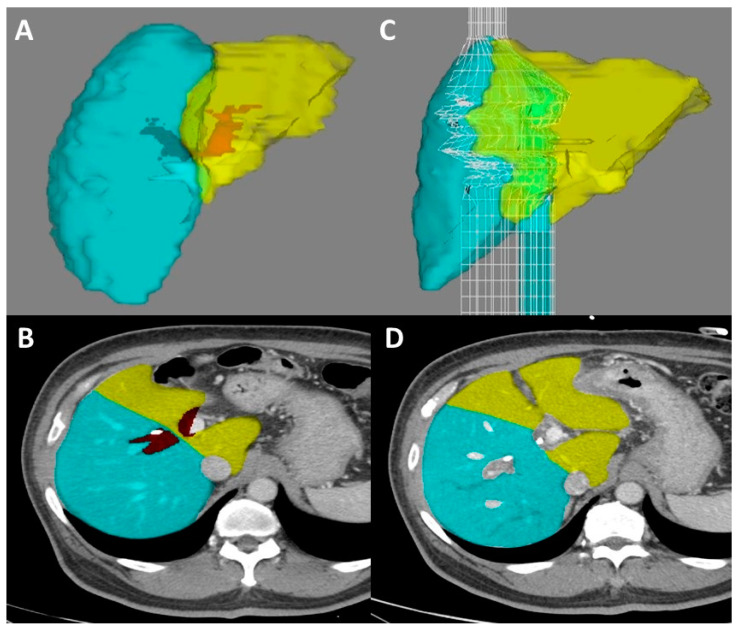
Preoperative and postoperative volumetric analysis with interval portal vein embolization. Computed tomography-based volumetric analysis (TeraRecon Inc., Durham, NC, USA) with perihilar cholangiocarcinoma. Prior to portal vein embolization (panels (A,B)), functional liver remnant after planned right hepatectomy was calculated to be 31.9% (yellow). Patient underwent right portal vein embolization without embolization of Segment IV. After three weeks, repeat volumetry was performed (panels (C,D)). The newly calculated FLR (yellow) was 45.9%, yielding a KGR of 4.7%.
